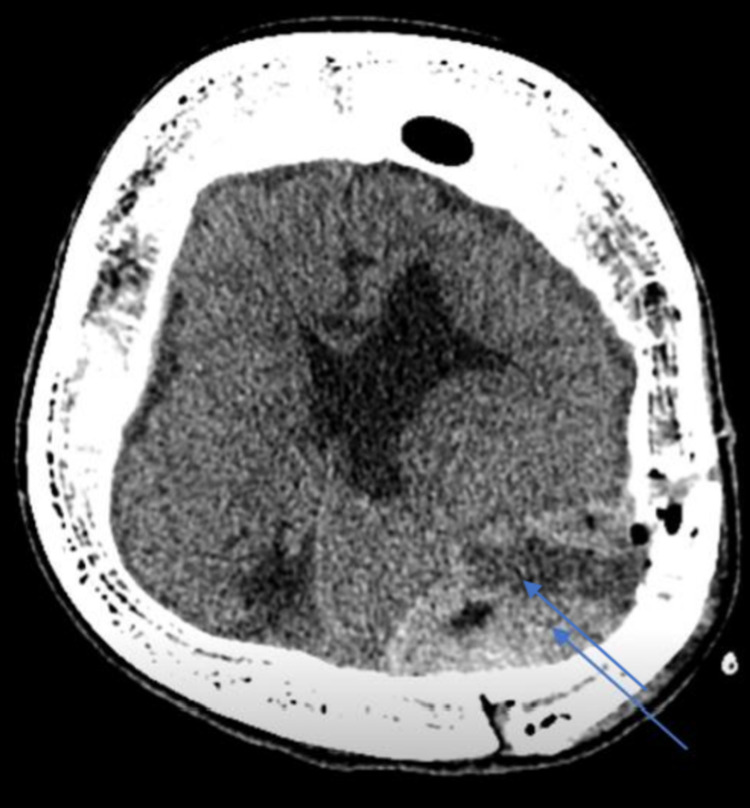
CT brain done three weeks postoperatively showed superficial collection beneath the surgical defect, extra-axial collection with an irregular enhancing rim measuring approximately 4.2 x 4 cm (maximum axial dimension) (pointed in the image).
